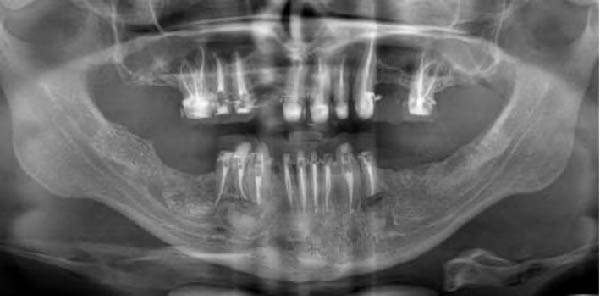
Follow‐up panoramic radiograph after 9 months showing bone formation at the sites of the lesions.
